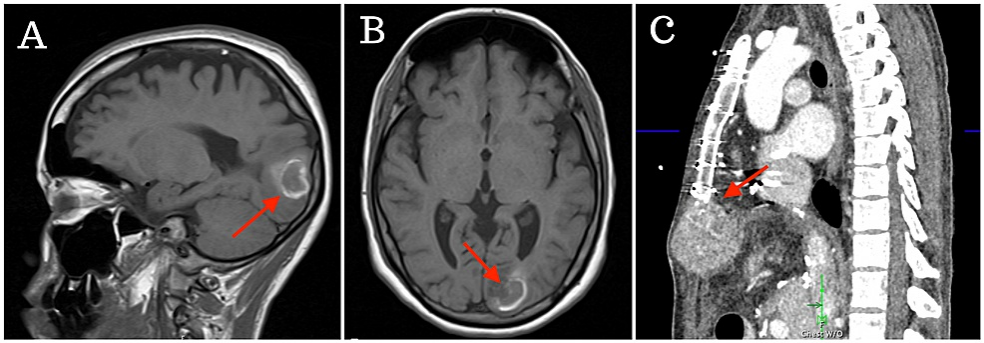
Imaging workup revealing metastatic HCC HCC, hepatocellular carcinoma (A) Sagittal view of MRI revealing a brain lesion consistent with hemorrhagic metastatic HCC; (B) axial view of MRI revealing an enhancing mass in the left occipital lobe; (C) CT of the chest and abdomen demonstrating a metastatic focus involving the lower sternum and xiphoid
